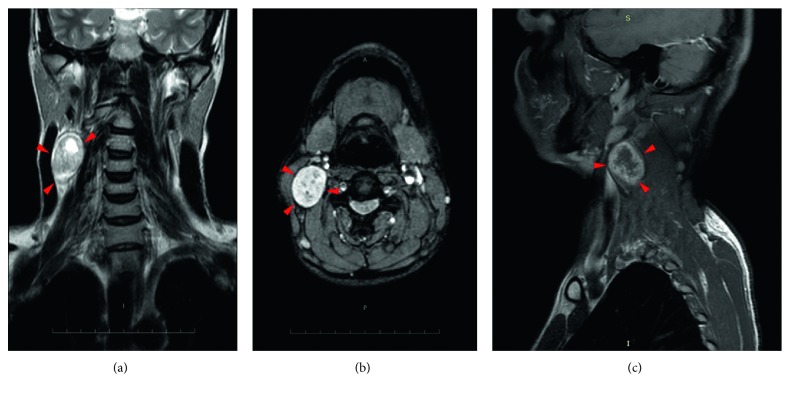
(a) T2-weighted coronal cut. (b) T2-weighted axial cut. (c) Postcontrast T1-weighted sagittal cut.
